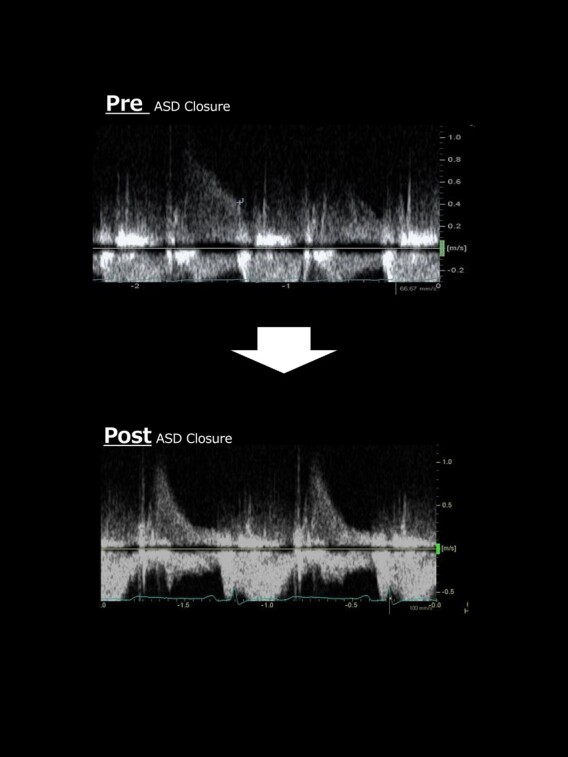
The change of continuous-wave Doppler waveform of the pulmonary regurgitation between before and after the atrial septal defect closure shows a steep decrease in pressure gradient suggesting an increase in diastolic right ventricular pressure.
